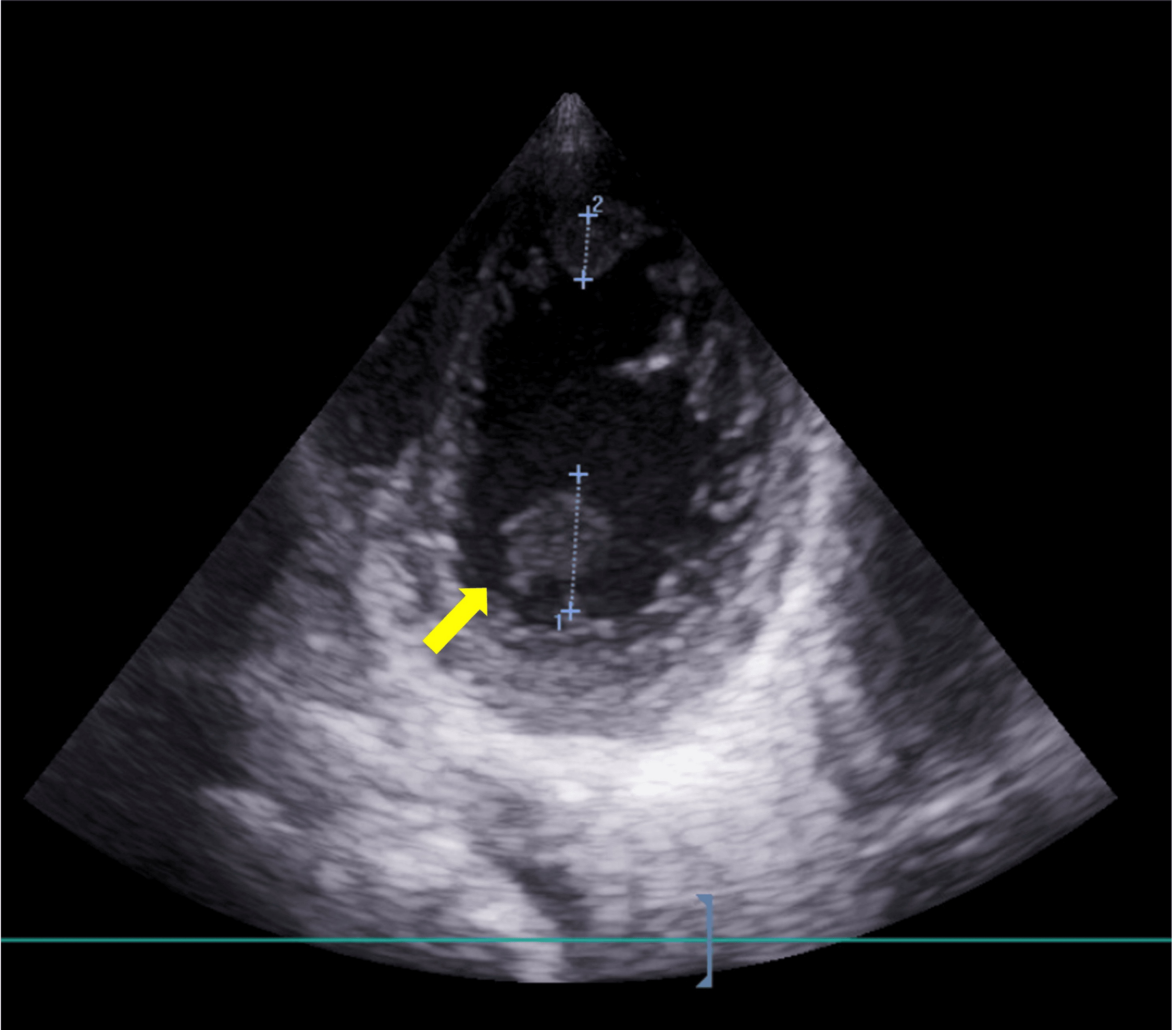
Transthoracic echocardiography image. Parasternal short-axis transthoracic echocardiography (TTE) image showing a mobile left ventricular thrombus (LVT) on day four of hospitalization (arrow) [7].
